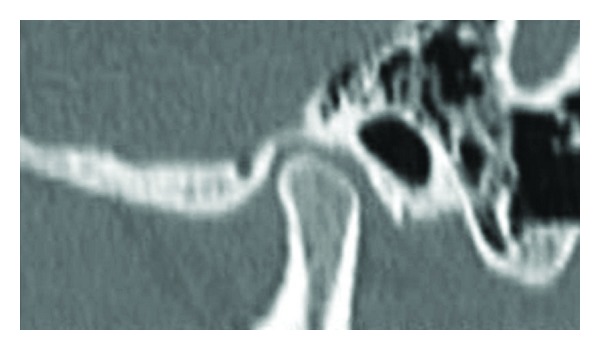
Sagittal slice computed tomography image of Craniomandibular articulation.
